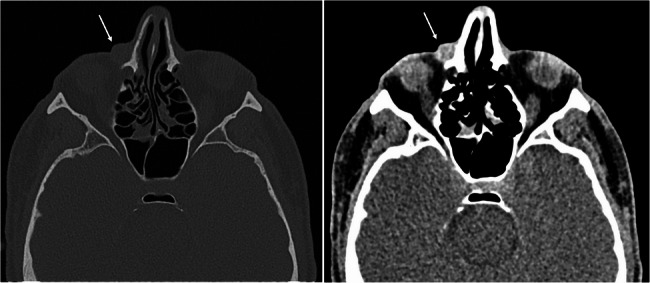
Facial computed tomography (CT) scan, showing a slightly hyperintense preseptal nodule (arrow) overlying the right medial canthal tendon, and slight air entrapment in the controlateral (left) lacrimal sac
